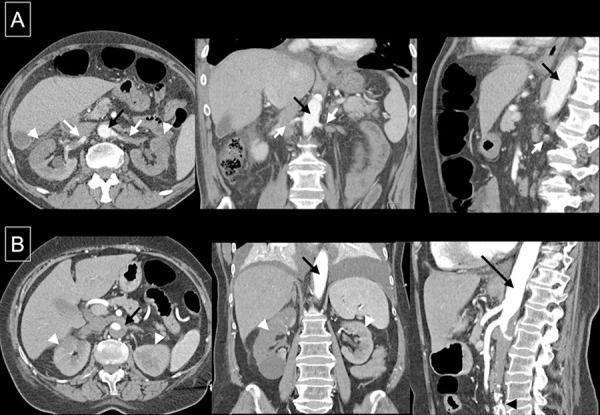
A: CTA images at diagnosis of Case 1. B: CTA images at diagnosis of Case 2. White arrows = renal artery; black arrows = aorta; white arrowheads = renal infarct; black arrowheads = aortoiliac stent.
